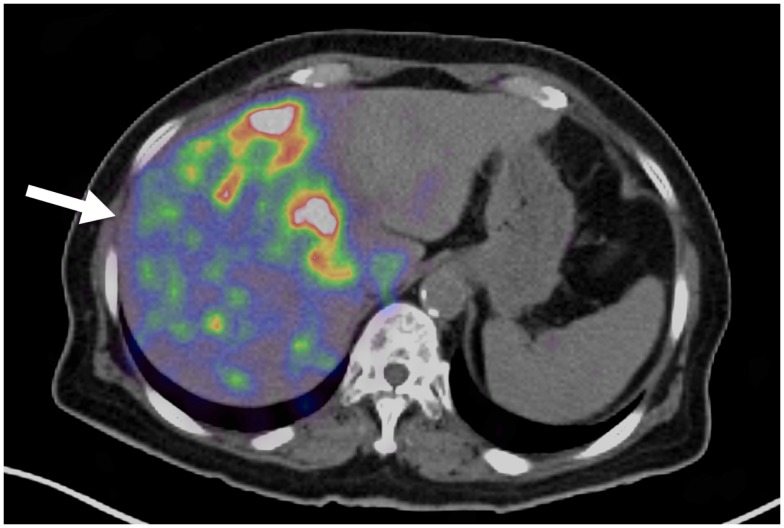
Yttrium-90 PET/CT following radioembolization. Note the non-uniformity present in the hepatic parenchyma (arrow).
